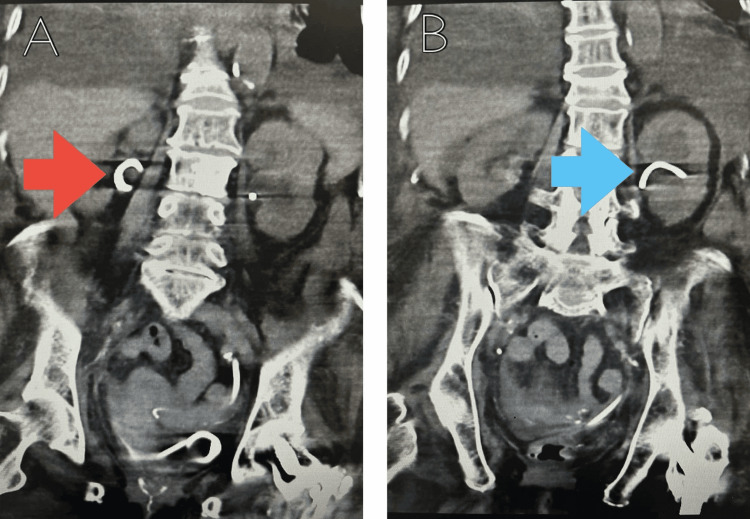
Coronal views of the CT scan of the urinary tract A: A coronal view of the CT scan of the urinary tract showing the right ureteric stent (red arrow). B: A coronal view of the CT scan of the urinary tract showing the left ureteric stent (blue arrow). CT: computed tomography
